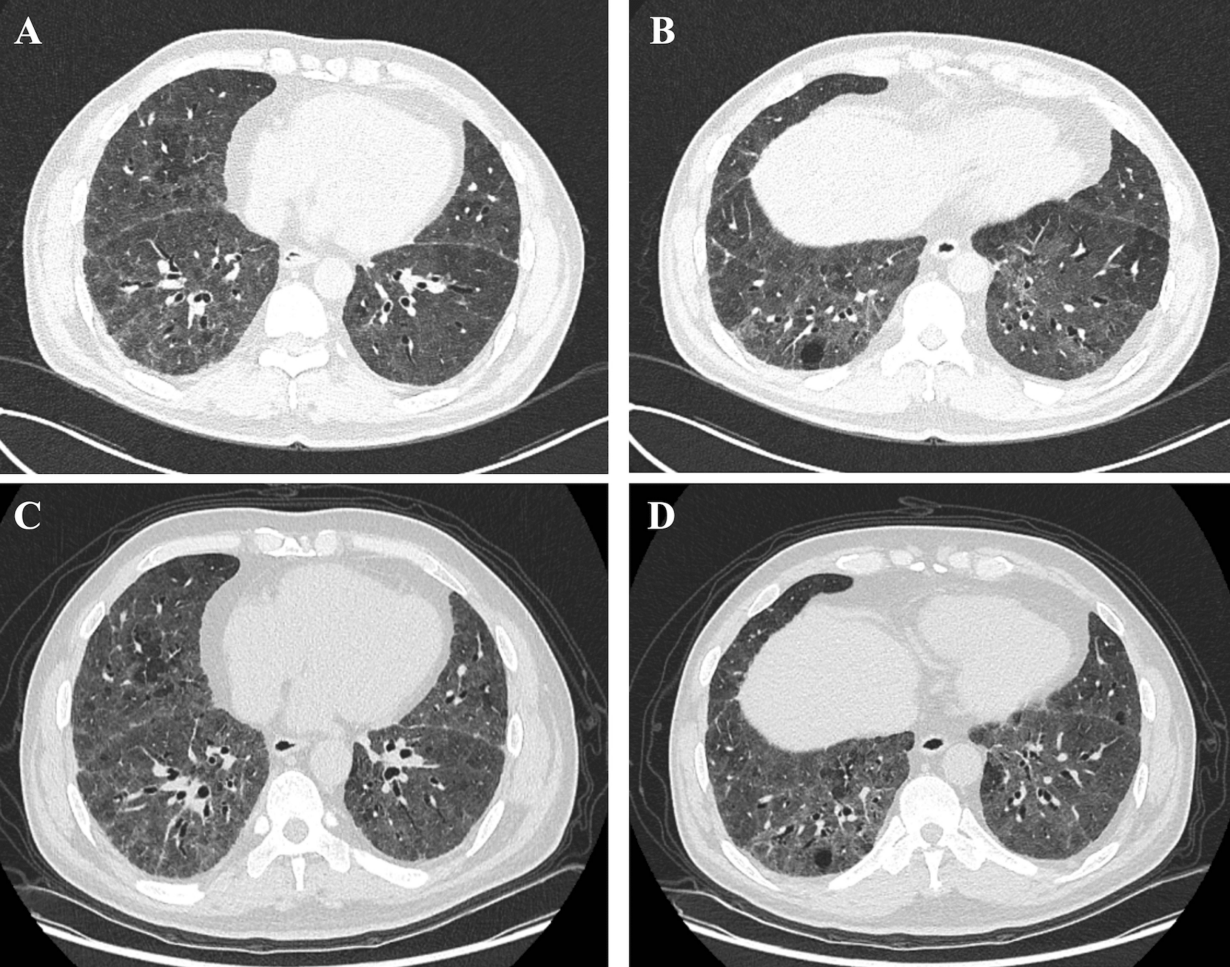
Post-treatment chest CT (A, B) Chest CT after avoiding exposure to chili powder for one month revealed a reduction in inflammation, although fibrosis persisted. (C, D) At the fifth-month follow-up, no significant changes in interstitial inflammation were observed in both lungs compared to the prior scan. CT: computed tomography
